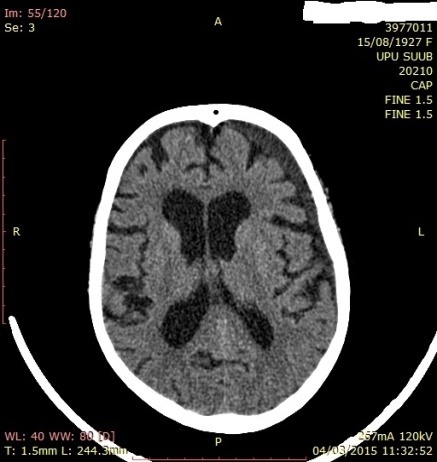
CT aspect with left F-T hygroma in patient P.M. of 87 years old on discharge and under neurosurgical surveillance
